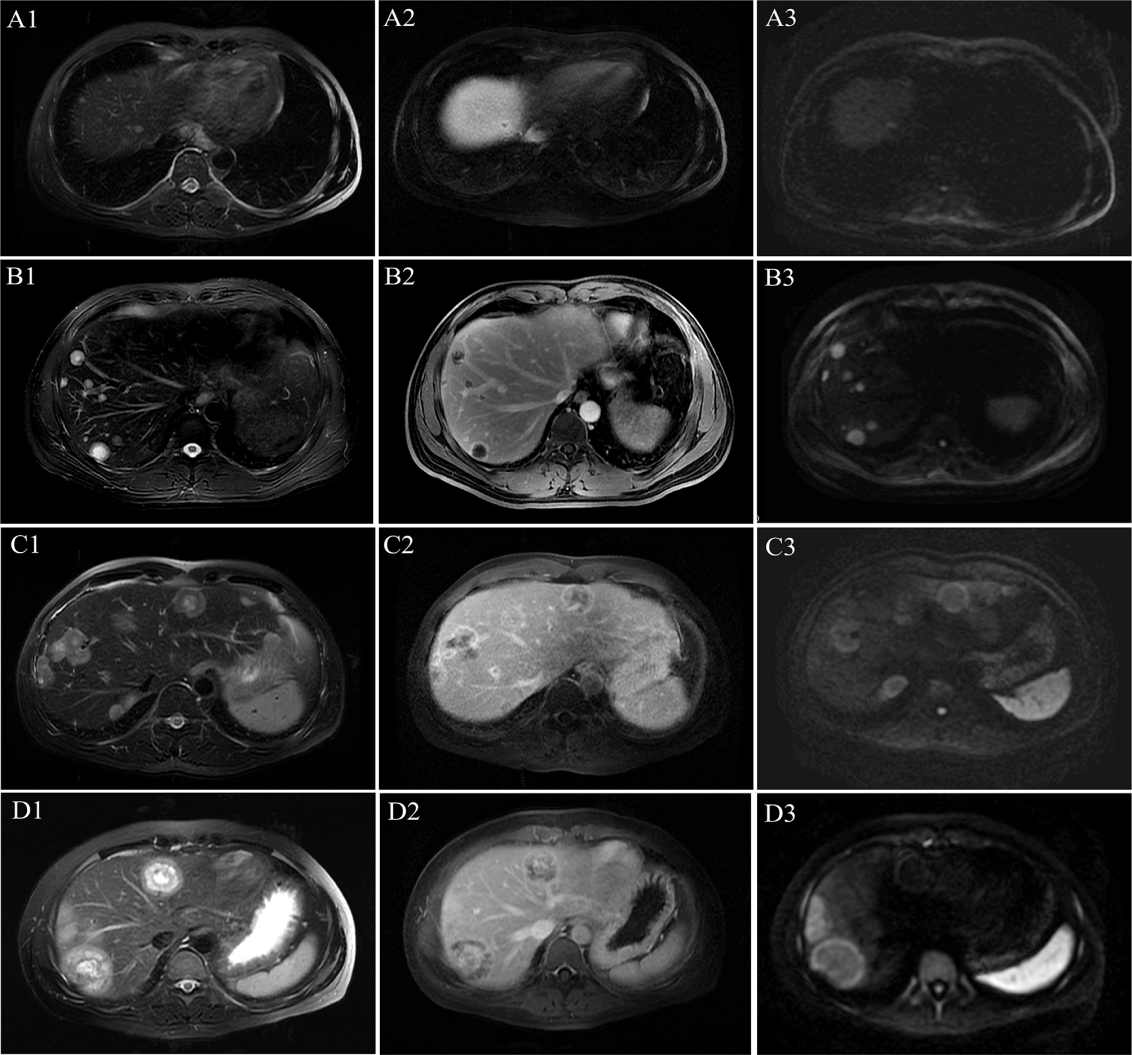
Multilayer ring appearance. The multilayer ring appearance was seen most clearly in T2-weighted imaging (T2WI). The multilayer ring appearance with alternating high and low signal intensity (except for in the single-layer structure). (A1–A3) MR images of lesions with a single layer in patient 1. (B1–B3) MR images of lesions with two layers in patient 1. (C1–C3) MR images of lesions with three layers in patient 5. (D1–D3) MR images of lesions with four layers in patient 4. (A1) T2WI shows a single layer with slightly high signal intensity. (A2) Single-layer ring appearance on the delayed phase with peripheral enhancement and low central signal. (A3) Single-layer ring appearance on the diffusion-weighted imaging (DWI) image shows slightly high signal intensity. (B1) T2WI shows a two-layer ring appearance with slightly high peripheral and high central signal intensity. (B2) Two-layer ring appearance on the delayed phase with peripheral enhancement and low central signal. (B3) Two-layer ring appearance on the DWI image shows high peripheral signal intensity and slightly high central signal intensity. (C1) T2WI shows a three-layer ring appearance with slightly high peripheral signal intensity (the first layer), high central signal intensity (the third layer), and intermediate (the second layer) signal intensity, which demonstrates lower signal intensity comparing with that of the other two layers. (C2) Three-layer ring appearance on the delayed phase with obvious peripheral enhancement (the first layer), low central signal intensity (the third layer), and intermediate (the second layer) slight enhancement, which was not as clear as on T2WI. (C3) Three-layer ring appearance on the DWI image shows peripheral hyperintensity (the first layer) and slightly high signal intensity for the other layers. (D1) T2WI shows a four-layer ring appearance with slightly high peripheral signal intensity (the first layer), high signal intensity in the adjacent second layer, and slightly high signal intensity in the third layer and high central signal intensity (the fourth layer). (D2) Four-layer ring appearance on the delayed phase with obvious peripheral enhancement (the first layer), hypo-enhancement in the second adjacent layer, and hyper-enhancement in the third layer with slight central hypo-enhancement, which was not as clear as those on T2WI. (D3) Four-layer ring appearance on the DWI image shows peripheral hyperintensity (the first layer) and slightly high signal intensity for the other layers.
